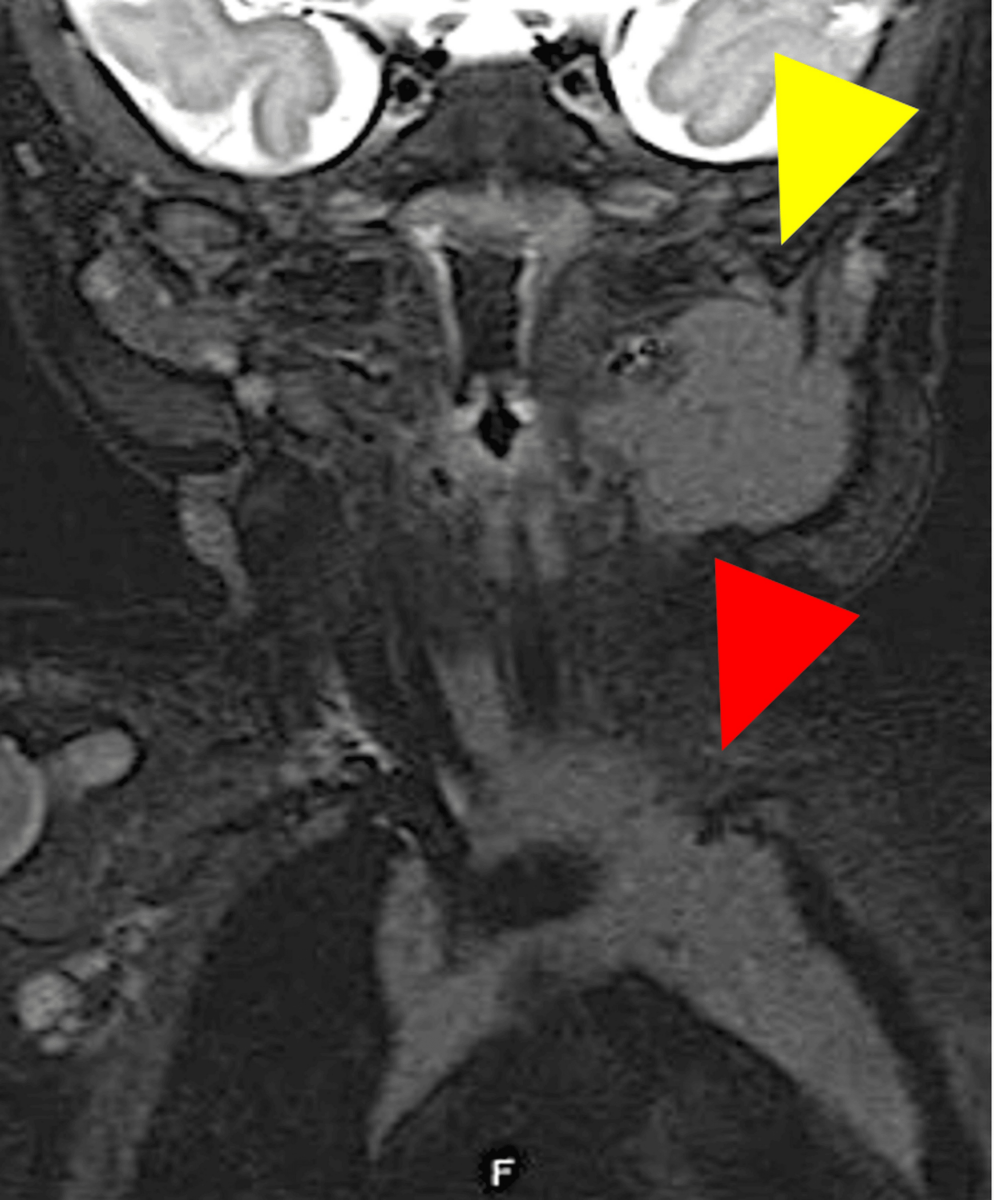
T2-weighted MRI of the cervical mass and the normal thymus T2-weighted MRI showing the cervical mass (yellow arrowhead) and the normal thymus (red arrowhead) with identical signal intensity. MRI: magnetic resonance imaging
